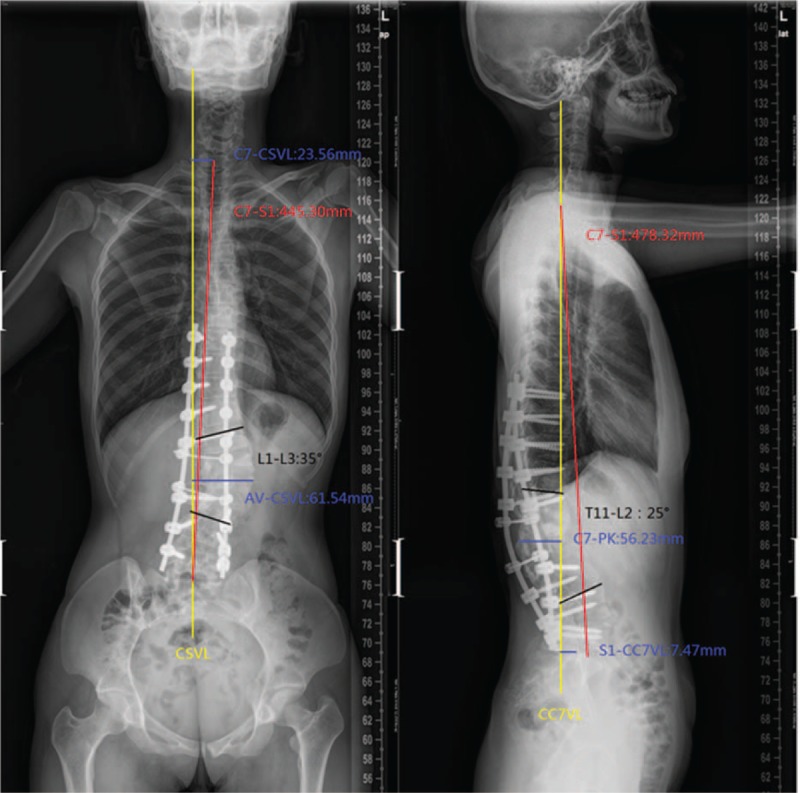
Lateral and anteroposterior X-ray, 3-month postrevision surgery follow-up.
